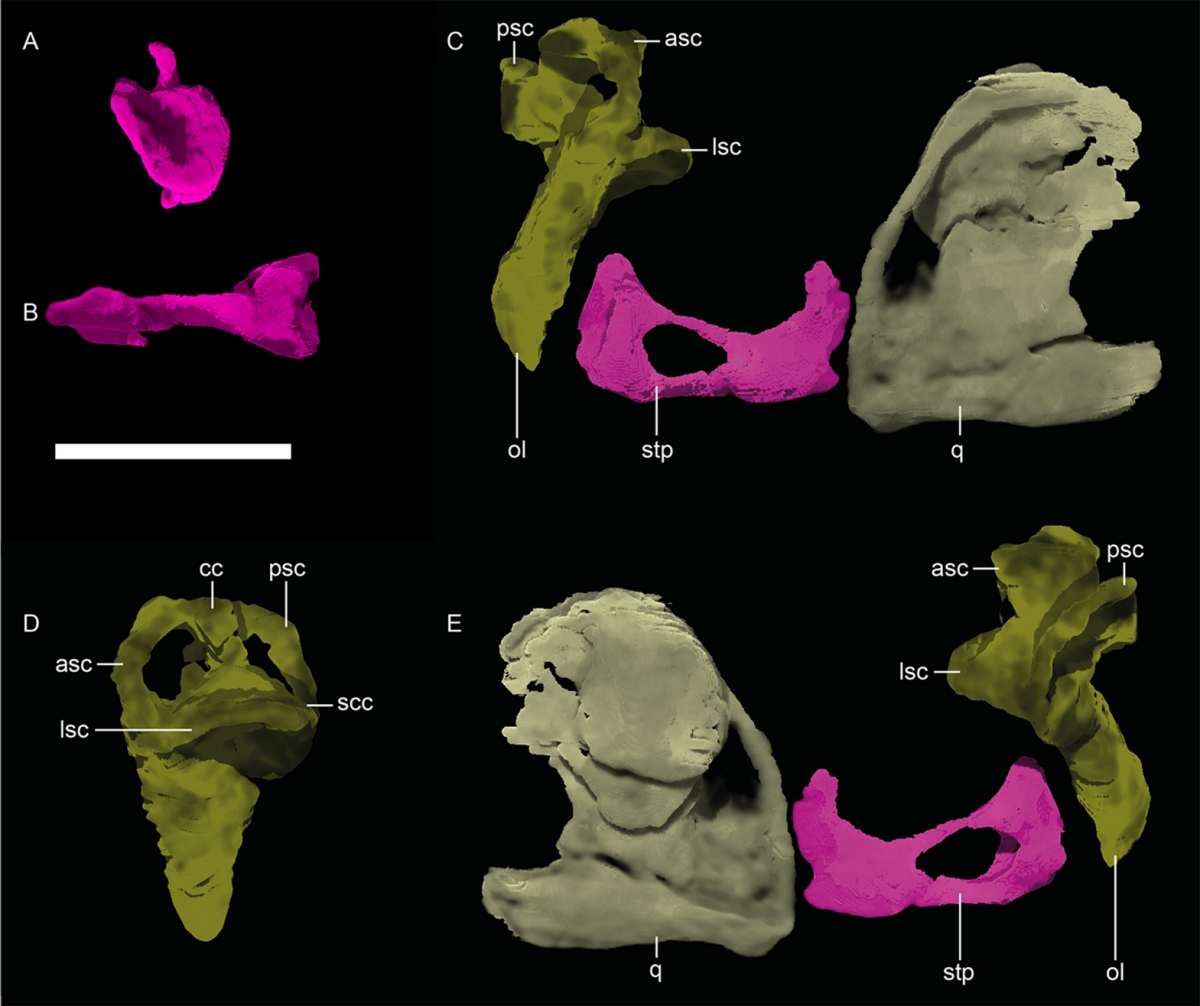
Neutron CT reconstruction of MB.R.999: stapes from (A) medial and (B) ventral; otic ear labyrinth from (C) anterior, (D) left lateral and (E) posterior. Abbreviations: asc, anterior semicircular canal; cc, common crus; lsc, lateral semicircular canal; ol, otic labyrinth; psc, posterior semicircular canal; q, quadrate; scc, secondary common crus; stp, stapes. Scale bar = 1 cm.
